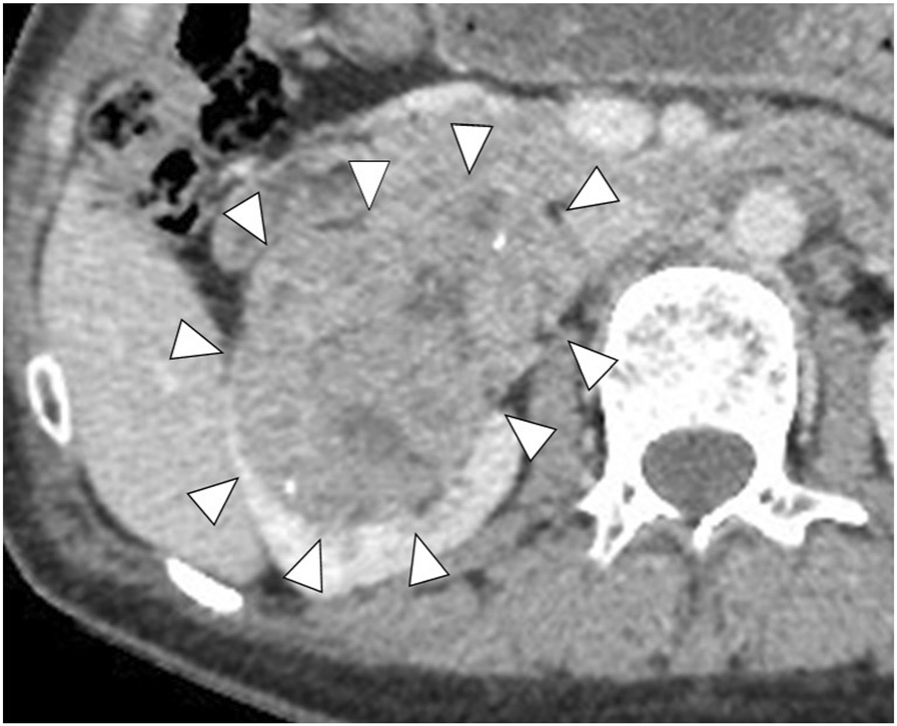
Enhanced abdominal computed tomography. Abdominal computed tomography revealed a hypovascular tumor, measuring 9 × 7 cm, in the upper pole of the right kidney (arrowheads).
